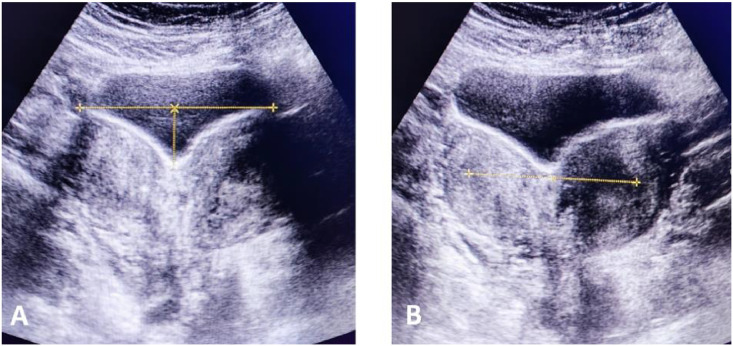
Coronal Transabdominal sonography (TAS) view of a bicornuate uterus and the deep intervening cleft between the 2 horns—(A) fundal cleft depth of 1 cm or more between the horns, (B) intercornual distance greater than 4 cm.
